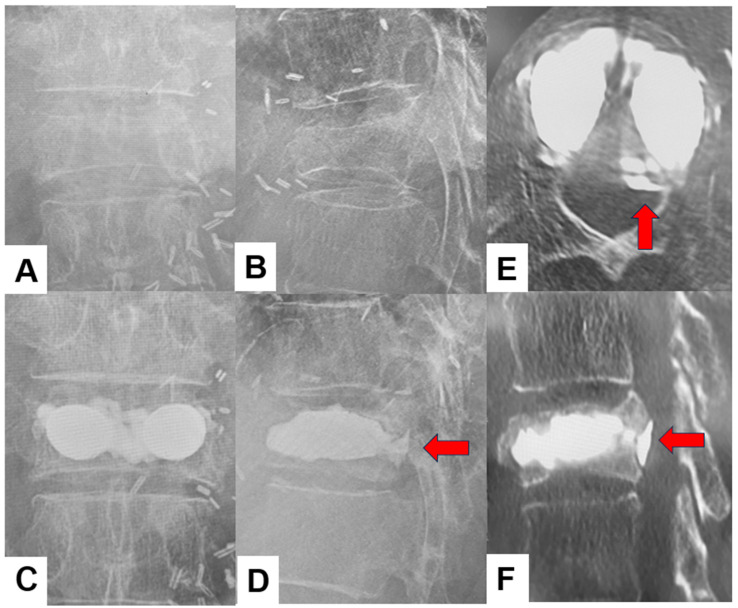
Cement leakage case of VBS, 84-year-old female of T12 OVF, (A): Preoperative anteroposterior radiogram, (B): Preoperative lateral radiogram, (C): Final follow-up anteroposterior radiogram, (D): Final follow-up lateral radiogram, (E): Axial CT, (F): Sagittal reconstruction CT. Red arrows indicate the cement leaked into the spinal canal.
